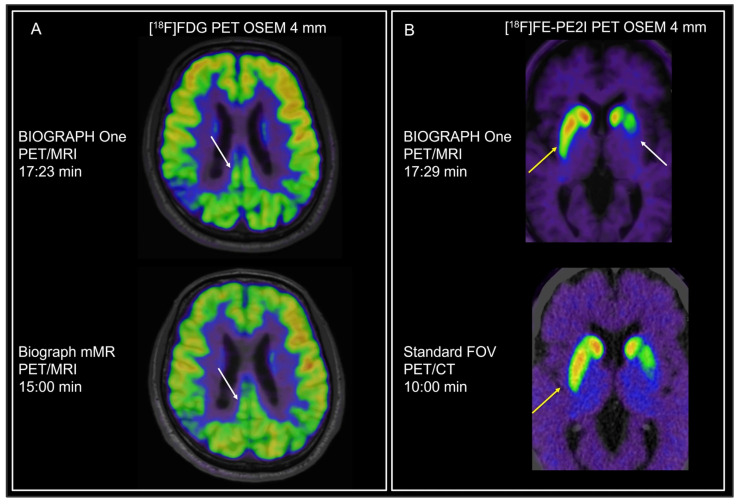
Neurodegenerative disease. (A) [^18^F]FDG PET imaging in a patient where Alzheimer’s disease was suspected. Imaging from both PET/MRI systems reveal marked parietal hypometabolism also involving the mesial parietal cortex and the cingulate gyrus typical of AD, more pronounced in the right hemisphere. Note the better delineation of the cortical structures on BIOGRAPH One PET/MRI (white arrows) at a similar duration of PET acquisition compared to Biograph mMR PET/MRI. (B) [^18^F]FE-PE2I imaging in a patient where Parkinson’s disease was suspected. Images show reduced dopamine transporter binding, predominantly in the posterior putamen and more pronounced on the left side corresponding to right-sided symptoms. MRI reveals no underlying structural pathology and supports primary nigrostriatal degeneration. The morphology could not be assessed on low-dose CT and warranted further imaging. Note also thinning of the posterior putamen on the right side not visually evident on PET/CT (yellow arrows), possibly because of higher resolution allowing better anatomical delineation or earlier identification of nigrostriatal dysfunction, or alternatively reflecting redistribution on late imaging.
